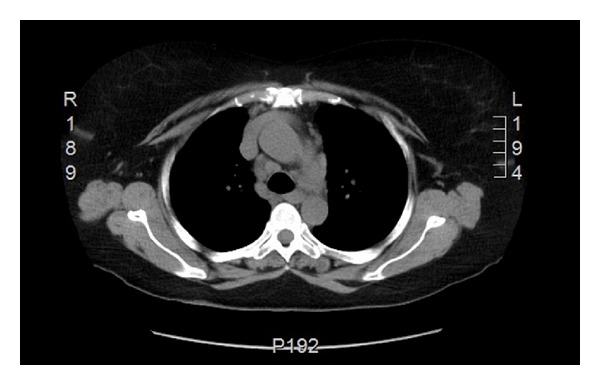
Follow-up thorax CT demonstrating resolution of mediastinal lymph nodes.
